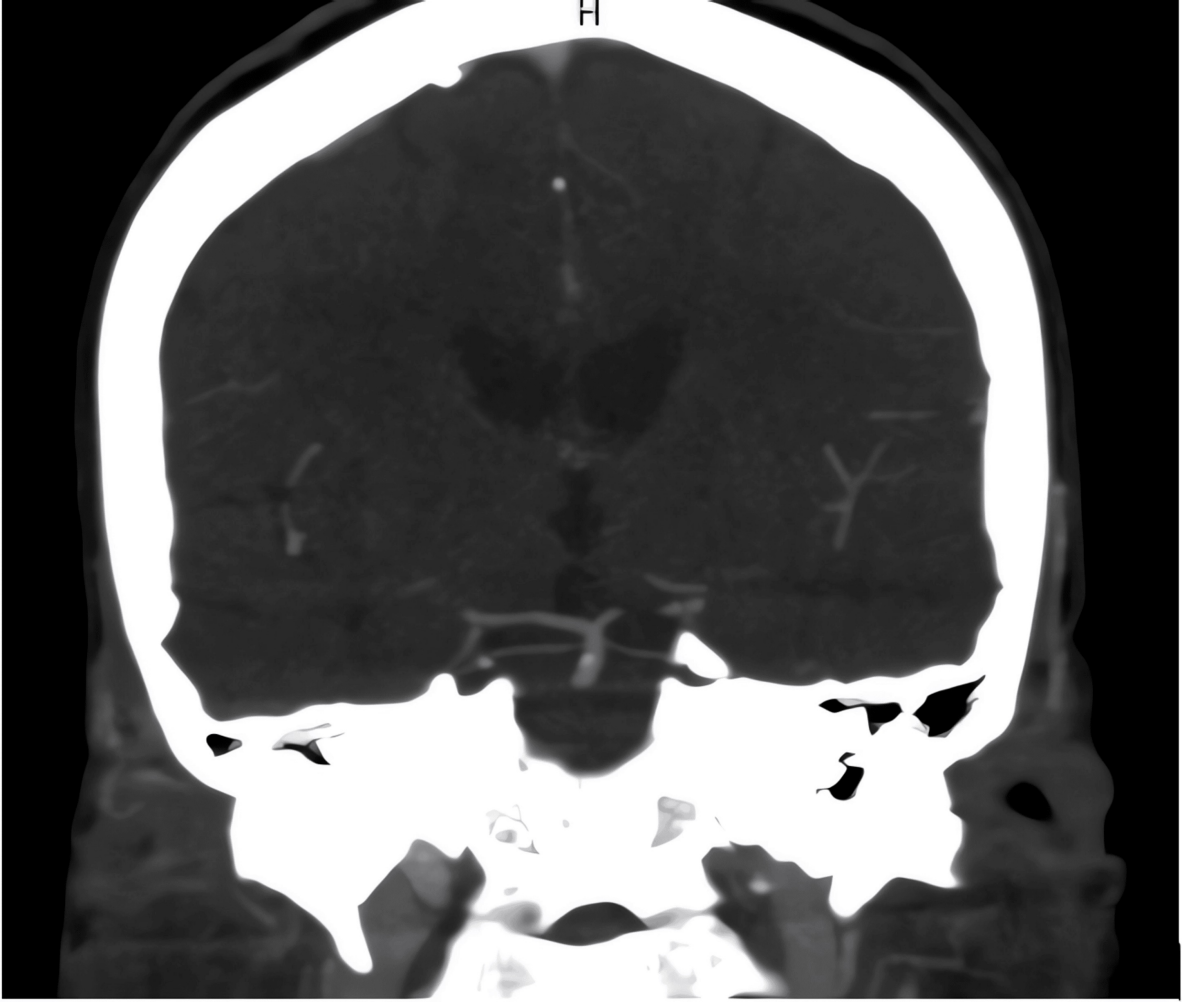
CT angiography demonstrating a proximal basilar artery cut-off, suggestive of an occlusion or high-grade stenosis.
